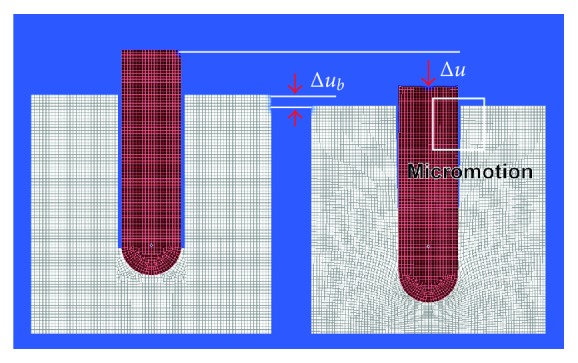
Without contact between implant and bone, an axial force acting on the implant causes implant dislocation as a result of elastic deformation of bone predominantly in the periapical region of the implant. Left: unloaded implant; right: loaded implant with implant displacement Δ*u* and displacement of cortical bone Δ*u*
